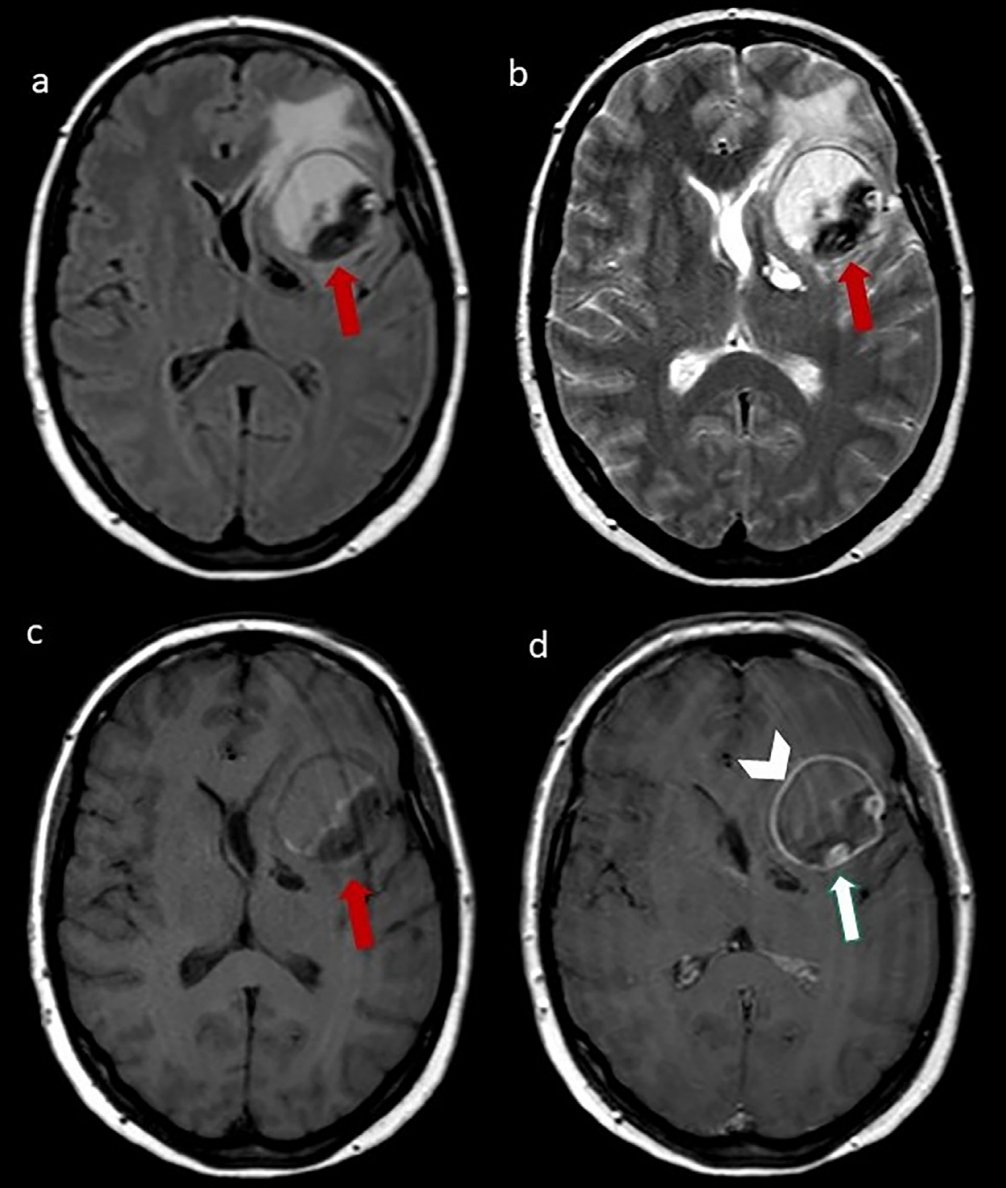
(A) Axial FLAIR, (B) axial T2- weighted image, (C) T1- weighted image, (D) T1- weighted postcontrastographic images showing a well-defined mass in the left frontal lobe characterized by inhomogeneous signal intensity on T1 and T2 weighted images, compatible with different stages of organizing hematoma, surrounded by perilesional edema. After contrast medium administration, an intralesional “serpentine” eccentric patent vessel (arrow) and a linear enhancement of the mass walls are evident (arrowhead).
